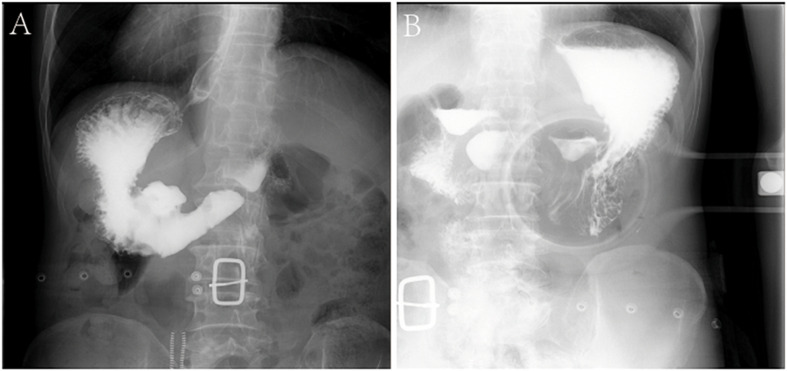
Characteristics of X-ray examinations of a 65-year-old male patient with GSC. (A,B) Reveals that there is a huge niche with irregular shapes at the small curvature of the stomach; the niche is located inside the outline of the stomach; the niche is surrounded by transparent bands with different widths, that is, ring embankments, with irregular outlines. The surrounding mucosa is thickened, interrupted, and the local gastric cavity is narrowed.
